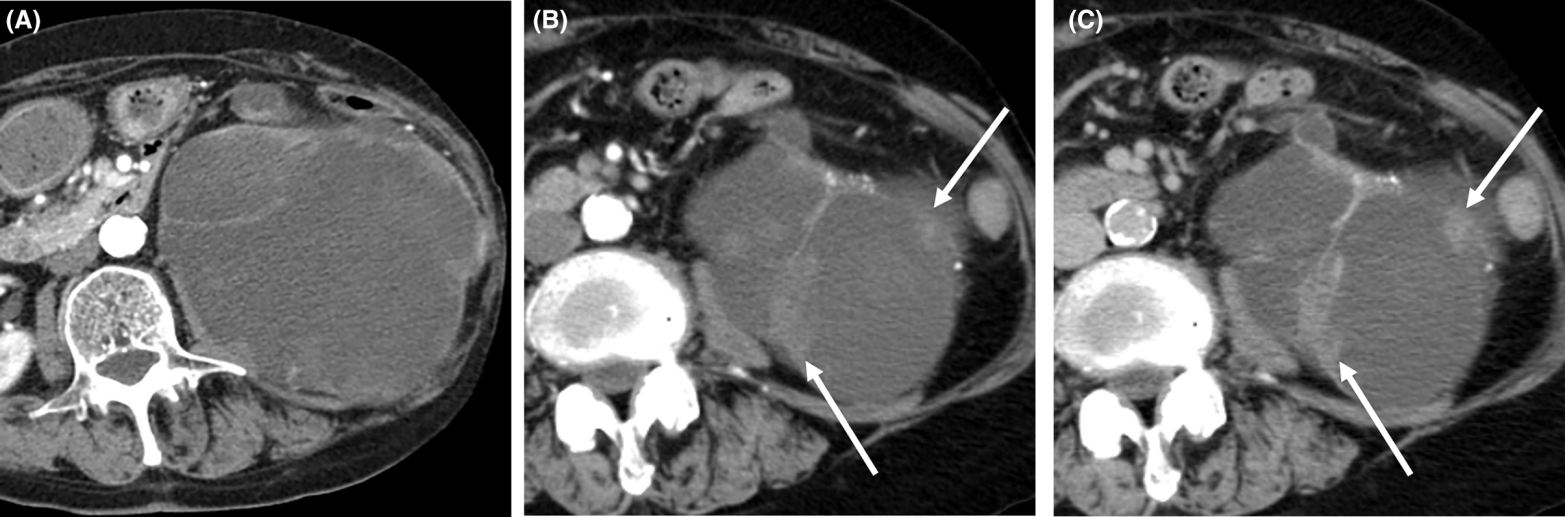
Contrast‐enhanced CT of the dilated renal pelvis and mural nodular lesions. The dilated renal pelvis is the main part of the lesion and renal parenchyma thinning (A). Irregular soft tissue parts with gradual enhancement at the wall of the dilated pelvis are shown arrows, (B) arterial phase; (C) delayed phase.
